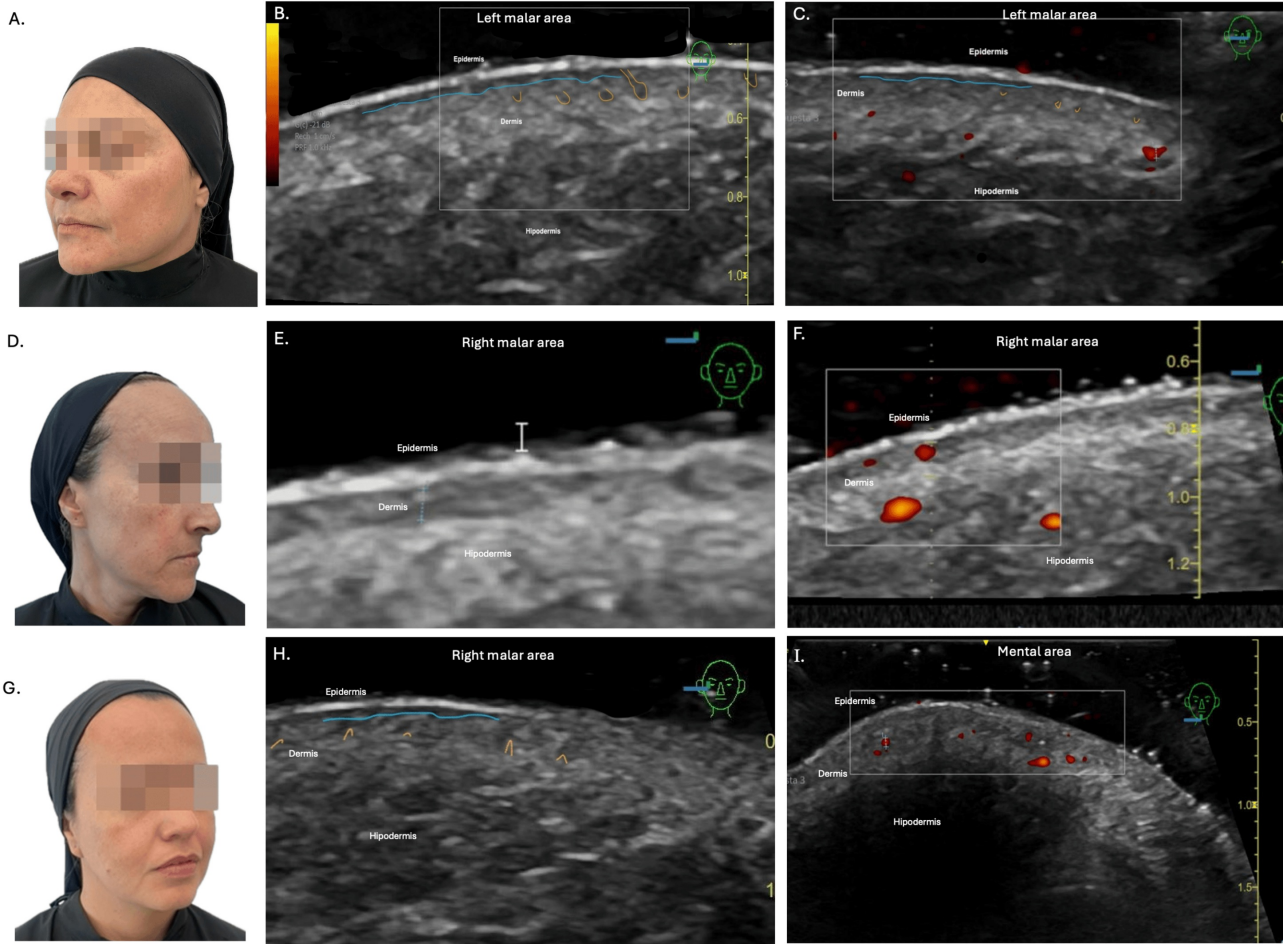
Initial clinical and high-resolution dermatologic ultrasound assesment A. Patient 1. Initial clinical evaluation. Mixed melasma predominantly in the forehead and cheek areas B. And C. Patient 1. Initial Ultrasound. Grayscale images: blue line: SLEB; yellow line: follicular dilatation. Power Doppler: red areas represent dermal micro-vascularization D. Patient 2. Initial clinical evaluation. Mixed melasma predominantly in the forehead and cheek areas E. And F. Patient 2. Initial Ultrasound. Grayscale images and Power Doppler: red areas represent dermal micro-vascularization G. Patient 3. Initial clinical evaluation. Mixed melasma predominantly in the forehead and cheek areas H. And I. Patient 3. Initial Ultrasound. Grayscale images: blue line: SLEB; yellow line: follicular dilatation. Power Doppler: red areas represent dermal micro-vascularization SLEB: subepidermal low-echogenic band
